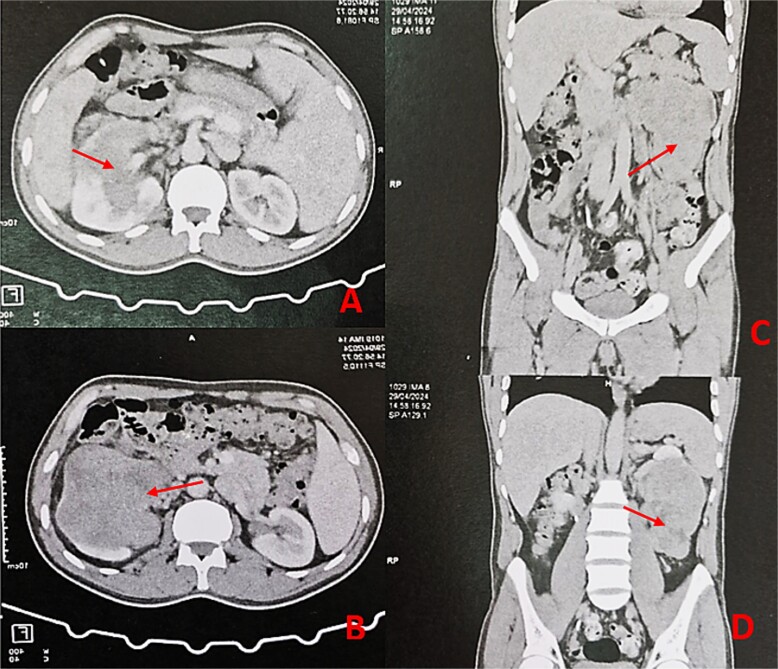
Thoraco-abdominopelvic CT scan with axial (A, B) and sagittal (C, D) images demonstrating a large heterogeneous mass (arrows) centered on the middle and lower thirds of the left kidney.
